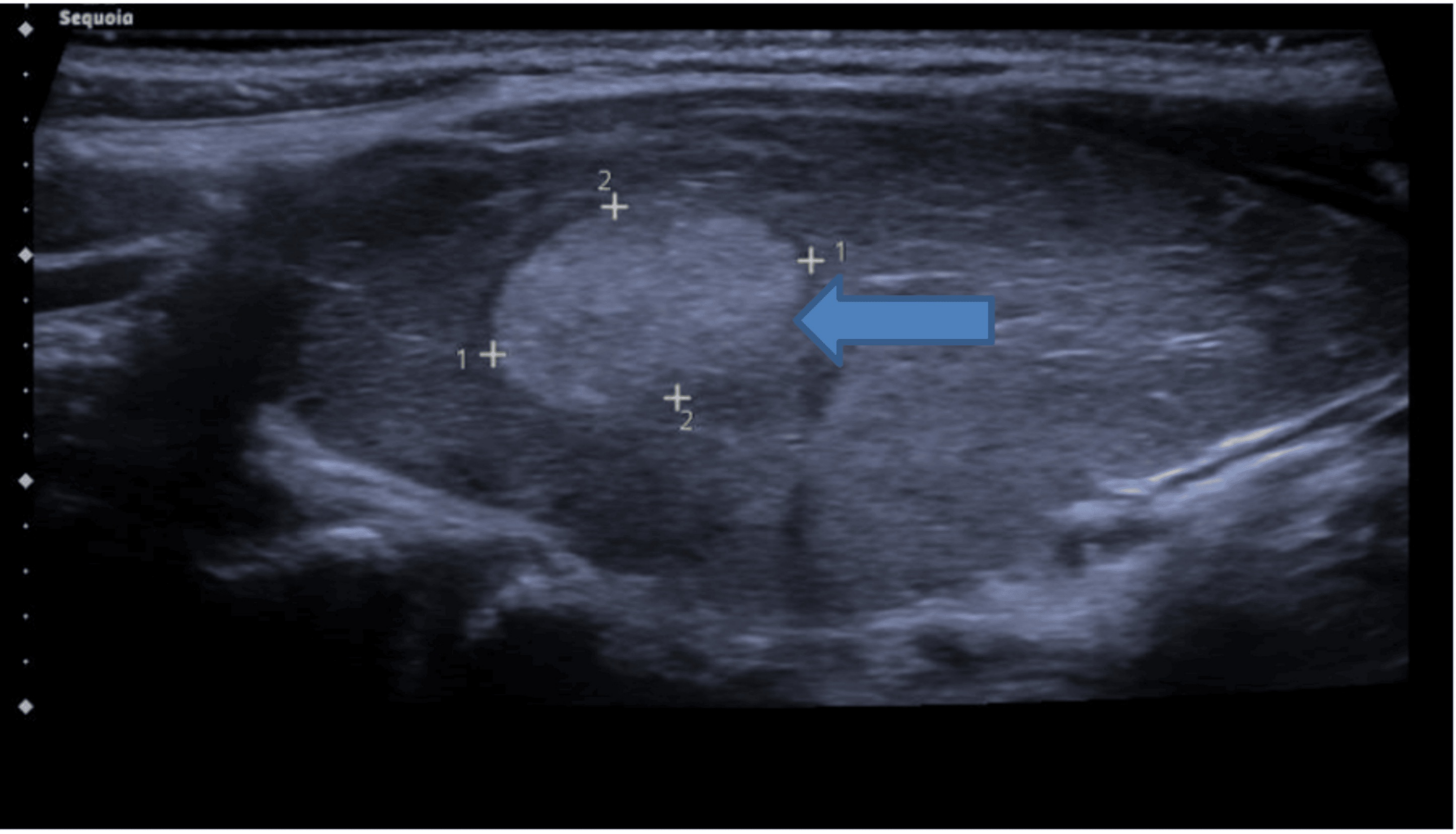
Ultrasound of the neck of the patient showing a single nodule
